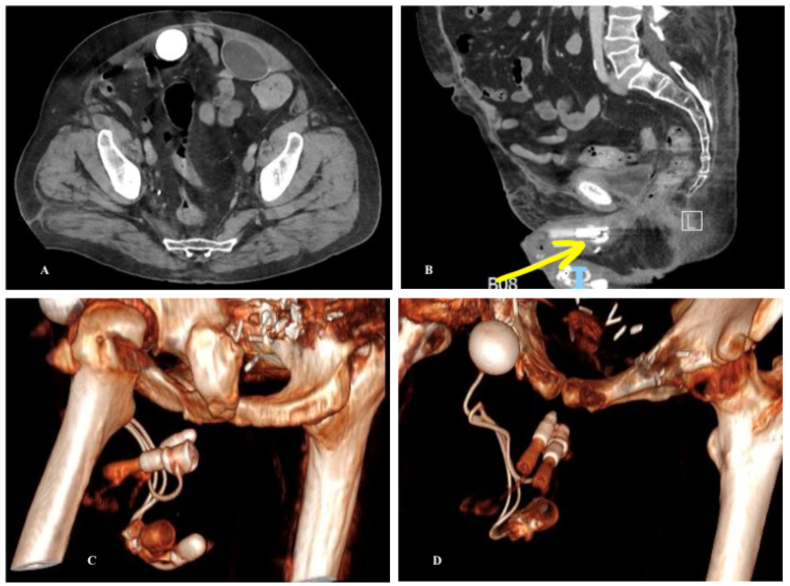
a. Post operative CT demonstrating prior AUS reservoir (on patient's right; filled with contrast) and recently placed sub-rectus IPP reservoir (on patient's left). b. Post operative CT demonstrating proximal end of the left IPP cylinder through the lumen of the transcorporal AUS cuff. c and d. 3D reconstruction demonstrating proximal end of the left IPP cylinder through the lumen of the transcorporal AUS cuff.
